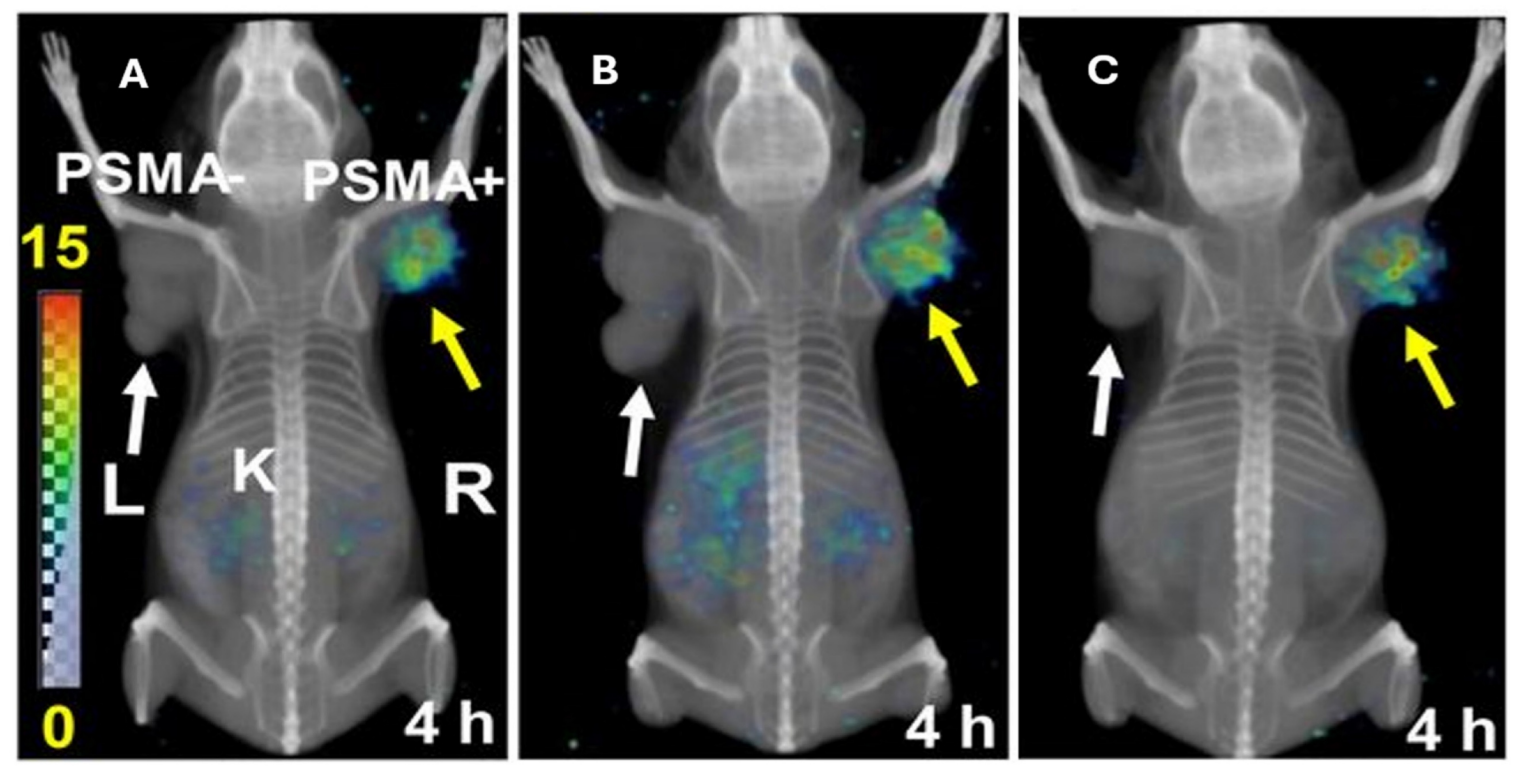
Whole-body volume rendered SPECT in PSMA+ (PC3 pip) and PSMA- (PC3 flu) tumors. The distribution of A) [^212^Pb]Pb-L2, B) [^212^Pb]Pb-L3 and C) [^212^Pb]Pb-L4 at 4 hours are depicted, with [^212^Pb]Pb-L2 showing the most optimal biodistribution for further evaluation (reprinted with permission from JNM. Banerjee et al., 2020,61:80-88. 93).
